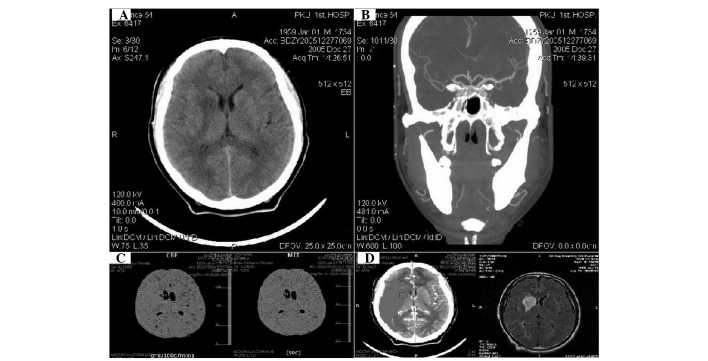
Case 1, male, 46 years old, with mixed aphasia combined with left limb weakness for 5 h. The patient was admitted to hospital and presented no changes in consciousness. (A) A plain computed tomography (CT) scan revealed that cerebral infarction occurred in the right basal ganglia, corona radiata and semiovale center, right temporal lobe and parietal lobe. (B) CT angiography (CTA) revealed that the first portion of the right middle cerebral artery was in severe stenosis. (C) Cerebral blood flow (CBF) in the area of the right brain lesion was significantly reduced; the CBF ratio was <0.2, indicating irreversible ischemia. The CBF ratio around the lesion core was >0.2, indicating the ischemic penumbra. The mean transit time (MTT) of the right brain lesions was extended. (D) An infarct size of infarct area was identified by magnetic resonance (MR)-weighted imaging at <7 days.
